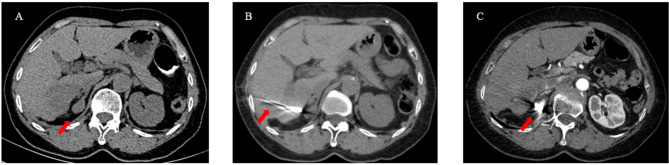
A 63-year-old woman with HCC oligometastases. (A) The CT scan showed HCC oligometastasis (red arrow) in the right adrenal gland; (B) Under the guidance of CT, iodine 125 seeds were implanted into the metastatic by a 18G needle and turntable implantation gun (red arrow); (C) The CT scan showed that the Iodine 125 seeds were evenly distributed (red arrow).
